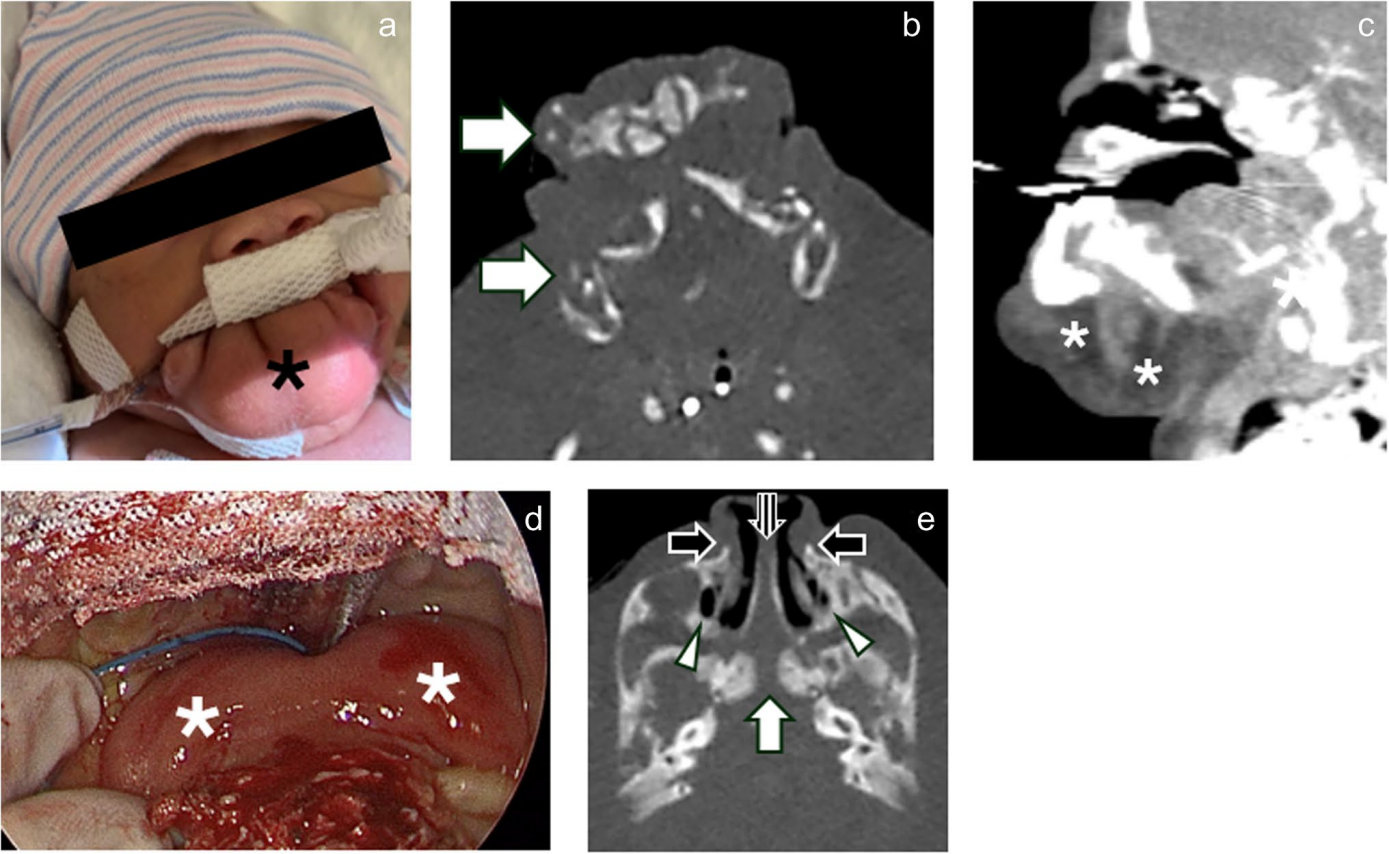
Craniofacial malformations. Clinical image (a) and axial (b) and sagittal (c) images of the face from a head CT angiogram demonstrate a skin-covered orofacial mass-like prominence (*black asterisk* in a) containing jaw-like elements that were anteriorly displaced from the dysplastic mandible (*arrows* in b) and excessive fat and soft tissue (*white asterisks* in c). Laryngoscopic image of the oral cavity (d) shows a bifid tongue (*white asterisks* in d). Axial oblique (e) image of the face and central skull base demonstrates a widened sella with a central osseous cleft (*white arrow*). Closed duplicated craniopharyngeal canals were associated with each of two pituitary fossae which were located parasagittally (*not pictured*). In addition, there is a widened pyriform aperture (*black arrows*), and duplication of the vomer (*striped arrow*) with widening of the nasal cavity. Each choana was duplicated, and the lateral choanal openings appeared blind ending (*arrowheads*). Laryngoscopic image is courtesy of Dr. Amy Whigham.
